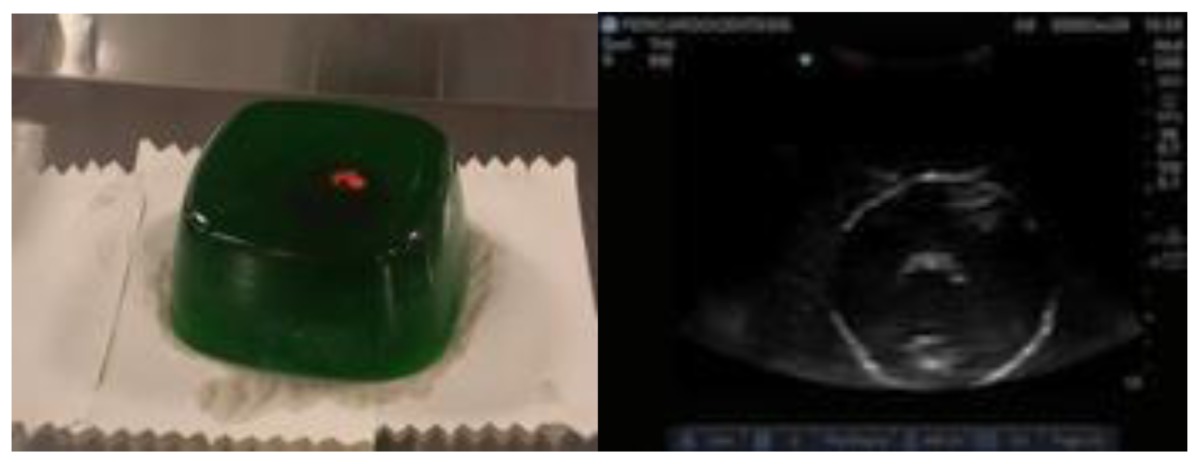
Ultrasound capable homemade pericardiocentesis model+ Approximate cost: $70/model
